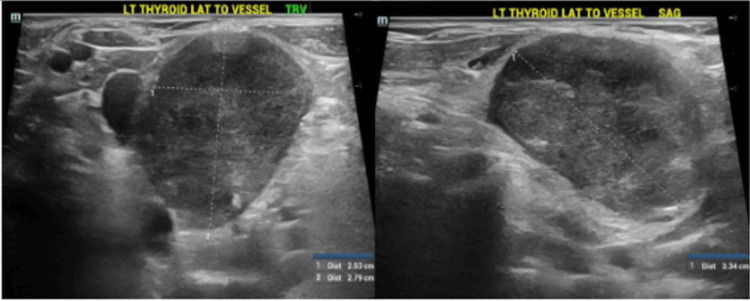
Ultrasonogram of the neck showing a 3.3 x 2.5 x 2.8 cm large solid mass besides the left thyroid lobe lateral to the internal jugular vein
